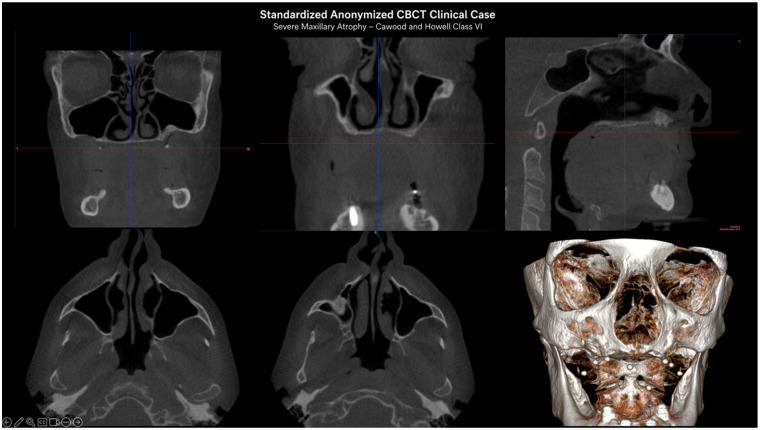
Standardized anonymized CBCT case used for treatment-planning assessment. Representative cone-beam computed tomography (CBCT) images of the standardized clinical case presented to all survey participants. The figure includes coronal sections at different anteroposterior levels, axial sections at different craniocaudal levels, a sagittal reconstruction, and a three-dimensional volume rendering. The images demonstrate severe maxillary atrophy consistent with Cawood and Howell Class VI and served as the radiological basis for therapeutic decision-making within the survey. All patient-identifiable information was removed prior to dissemination.
